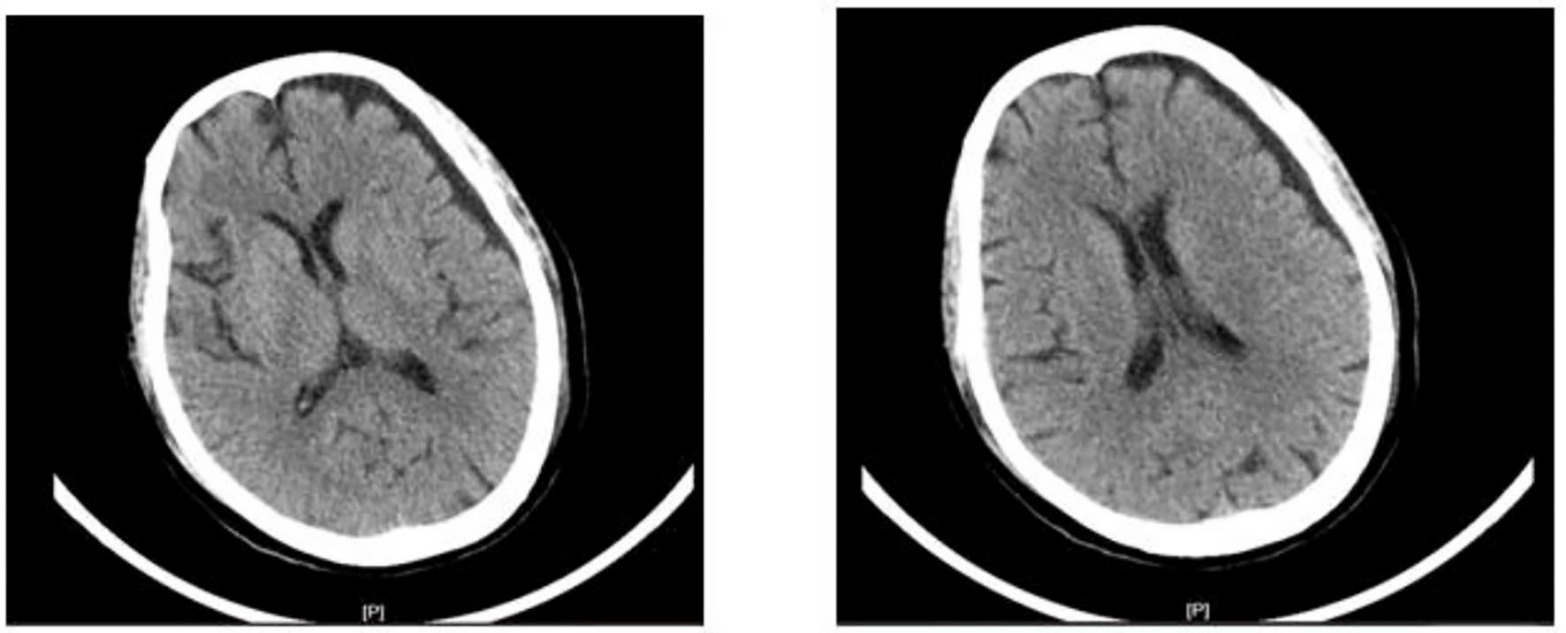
The computed tomography image of the brain on day 12.
